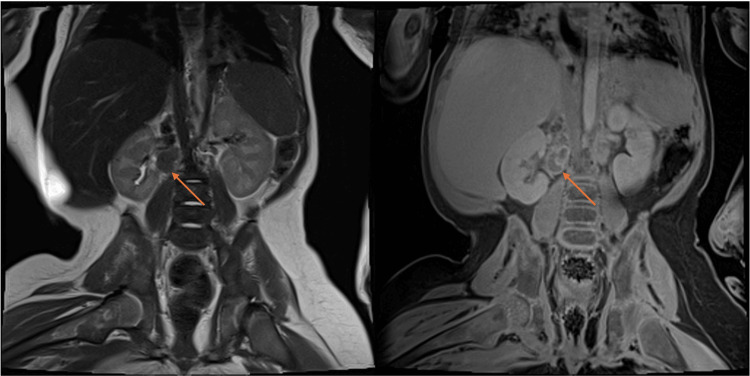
Post-treatment MRI: coronal T2 (left) and coronal T1 post-contrast on the right demonstrate positive treatment response and decreased size of the mass (orange arrows).
